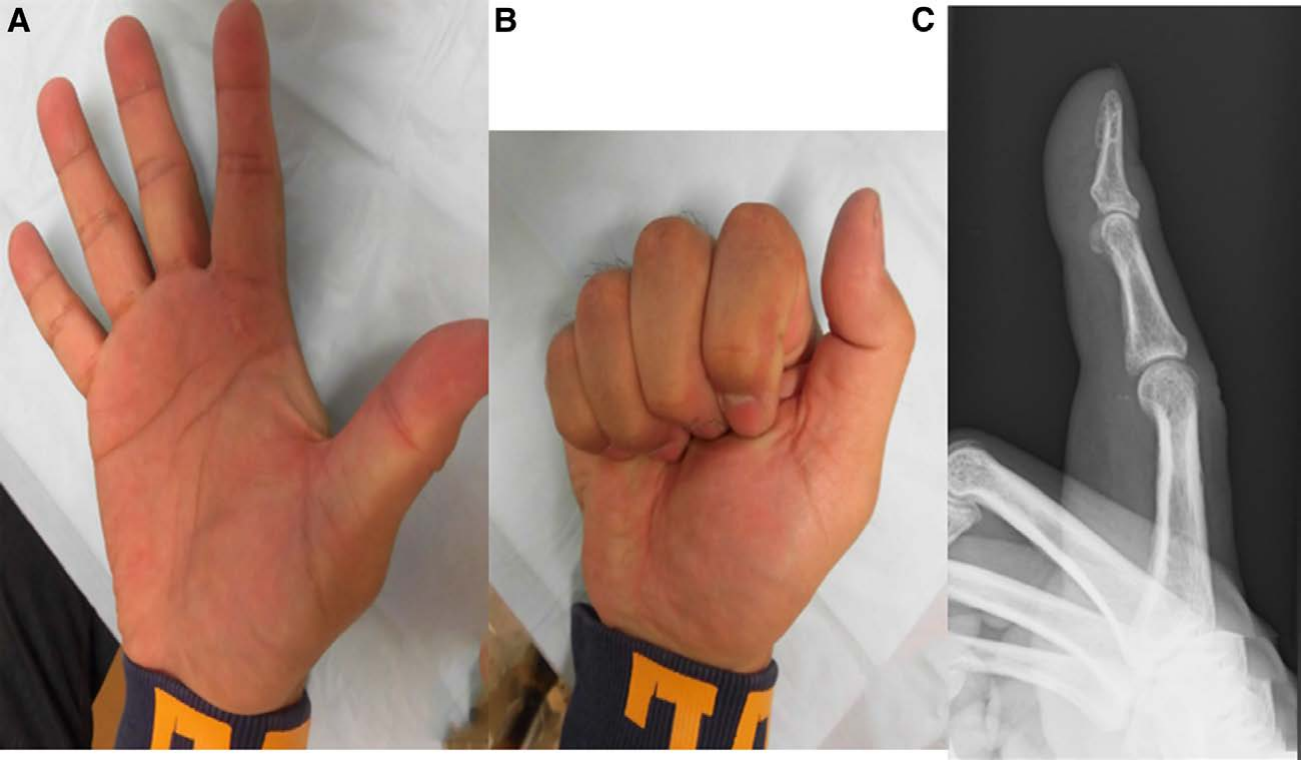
Clinical photographs of the hand after surgery. (A) Active extension. (B) Active flexion. (C) Postoperative radiography.
